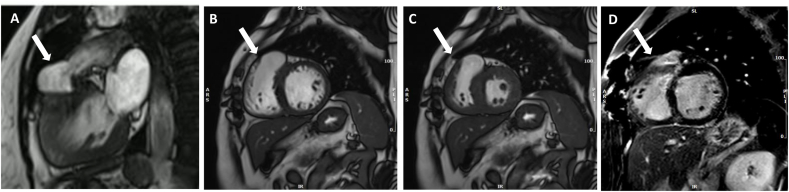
Cardiac magnetic resonance imaging (MRI) showing a showing a sizeable, aneurysmatic region of 28mm at the right ventricular outflow tract (A: sagittal plane, B, C: coronal plane). Myocardial late gadolinium enhancement (LGE) was observed in all surgically affected areas and the RV outflow tract dyskinetic region (D).
